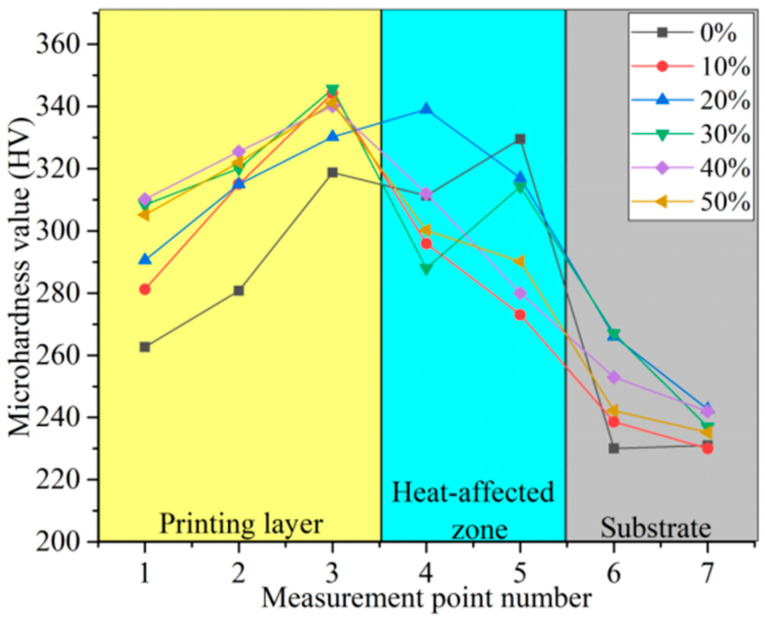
The microhardness values with different ultrasonic amplitudes (power ratios).
